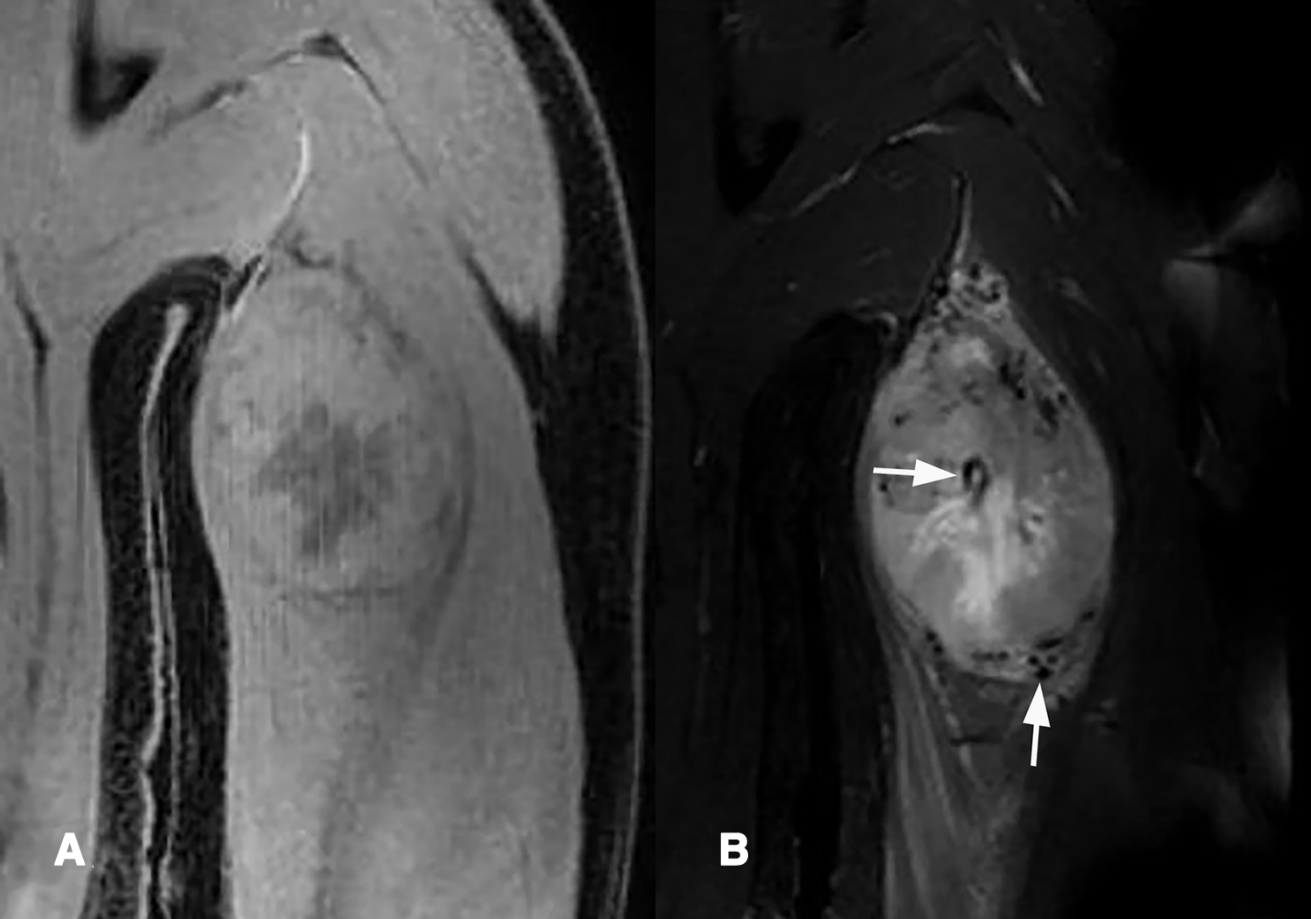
Preoperative MRI of an alveolar soft part sarcoma in the triceps brachii muscle. (A) Coronal T1-weighted image shows an moderate-signal intensity. (B) Coronal T2-weighted fat-suppressed image shows heterogeneously hyperintense and had flow voids that were both central and peripheral (arrows). MRI = magnetic resonance imaging.
